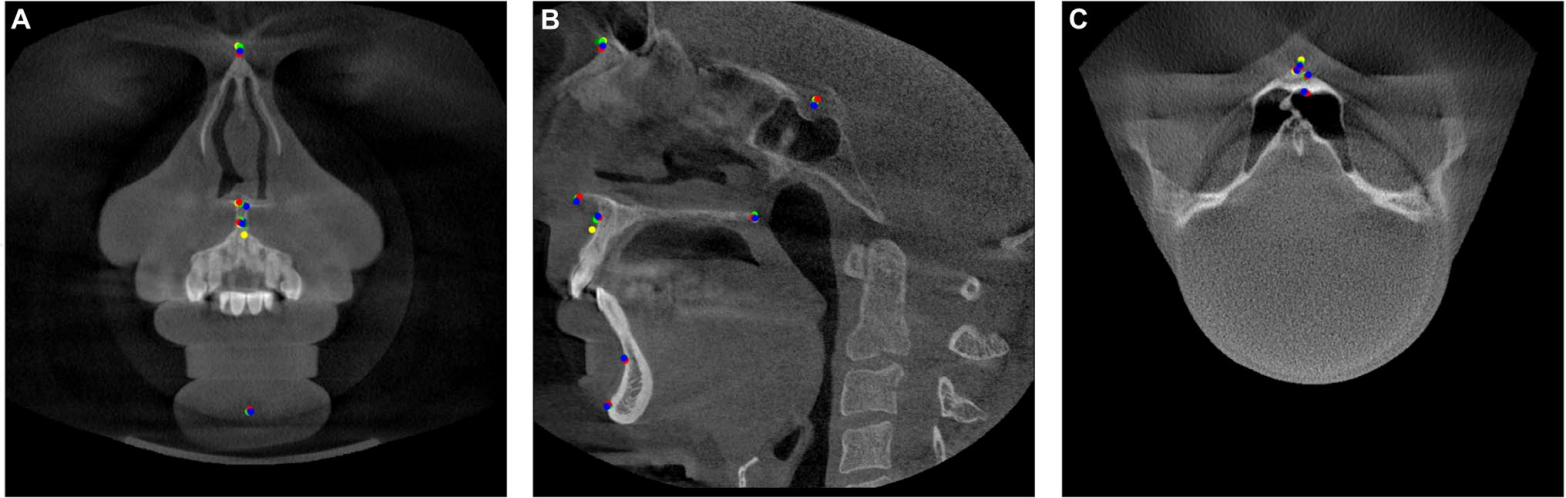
Sample of a CBCT image with median landmarks in coronal (A), sagittal (B), and axial (C) MPR view; red landmarks: GT; lime, green, yellow landmarks: experts 1, 2, 3; blue landmarks: AI
